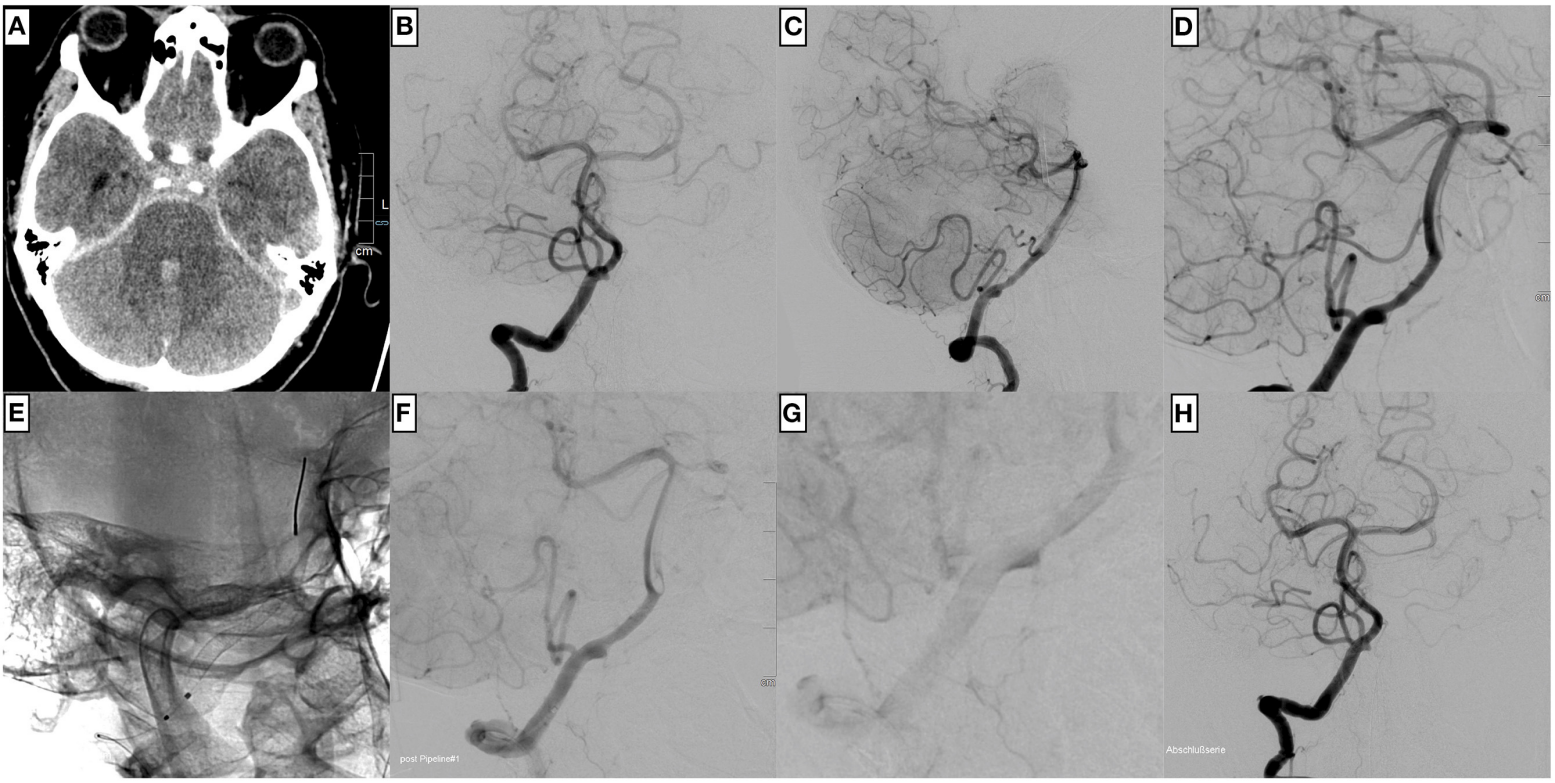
An example of uncomplicated PED implantation for treating a ruptured, dissecting aneurysm of the right dominant intradural vertebral artery in a 57-year-old male patient. (A) Non-enhanced cranial computed tomography shows Fisher grade 3 subarachnoid hemorrhage. (B) The injection of the right vertebral artery in posterior–anterior, (C) lateral, and (D) working projection demonstrates the comparatively confined ruptured dissecting aneurysm close to the posterior inferior cerebellar artery (PICA) orifice. After unimpeded catheterization with a Phenom™ 27, a PED (E) is implanted. The flow diverter is centered over the dissecting aneurysm. The control injection (F) shows the reconstruction of the vessel, now without irregularities in the post-PICA segment, which were apparent before implantation (D), and significant stasis of contrast agent within the aneurysm (G). The final angiogram in posterior–anterior projection (H) reveals timely opacification of the posterior circulation.
